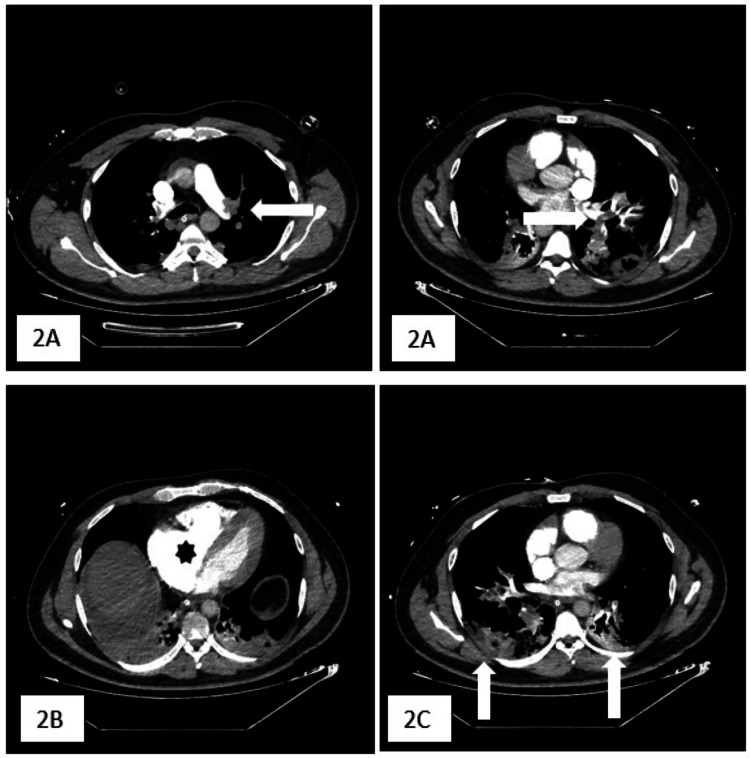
(A) Pulmonary artery computed tomography angiography confirmed extensive bilateral pulmonary embolism (horizontal white arrows) with (B) signs of right ventricular strain (black asterisk) and (C) pulmonary infarctions in the lower lobes (vertical white arrows).
